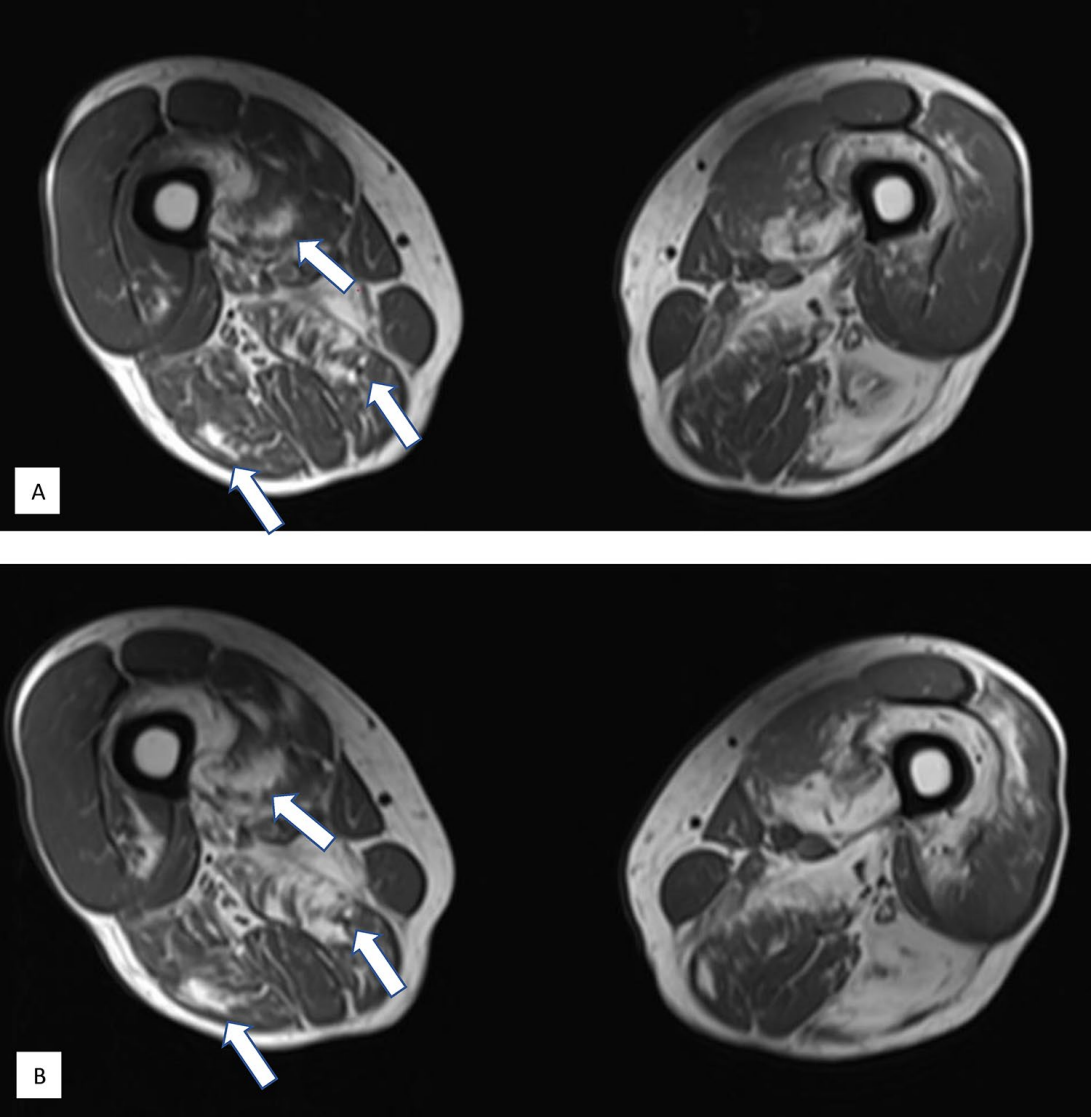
Representative example of progression from baseline in muscle fat replacement of thigh muscles in a 76-year-old (at baseline) male patient with a phenotype of mixed weakness. Axial T1 images at baseline (A) and follow-up (B). MRI reveals fat replacement, especially of the vastus intermedius, vastus medialis, and the posterior compartments of the thighs. Fat replacement increases from baseline to follow-up (arrows show examples). The patient did not have any attacks of paralysis during the follow-up period
